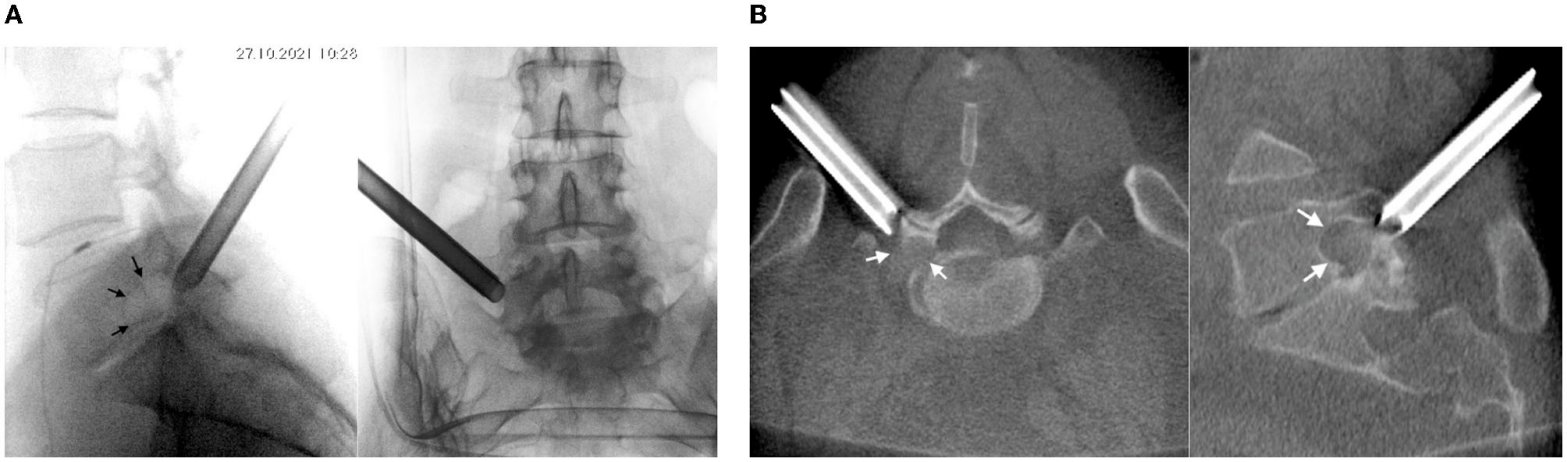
Intraoperative (A) X-rays and (B) CT (O-arm) of the position of the working tube at the left intervertebral foramen L5-S1 (see arrows).
